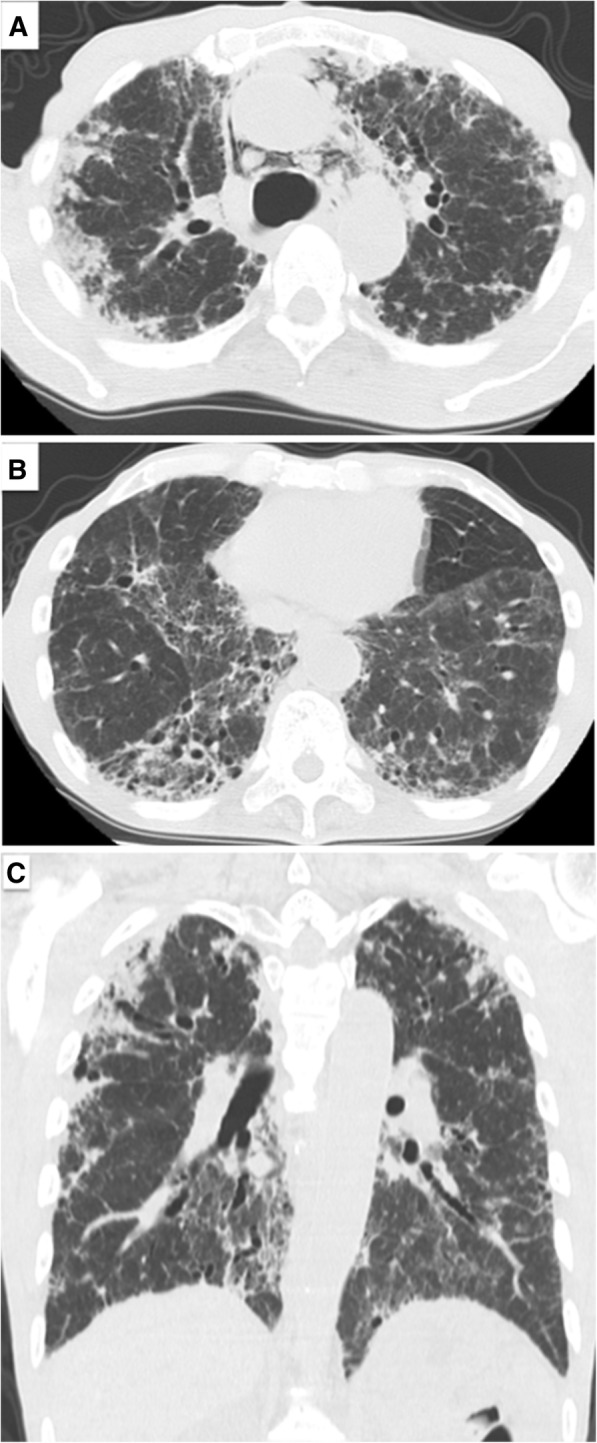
High-resolution computed tomography showing severe bilateral pleural thickening with shrunken and distorted upper lobes (a, c). There was mediastinal emphysema (a). On the other hand, reticulonodular shadows and bronchiectasis were distributed deep inside the lower lung (b, c)
